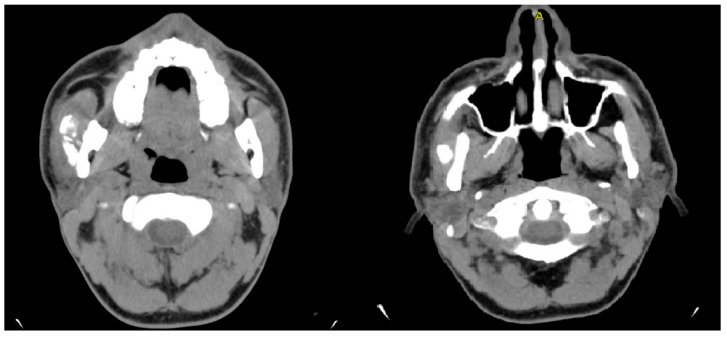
Axial CT images revealing a well-defined calcified mass within the right masseter muscle.
